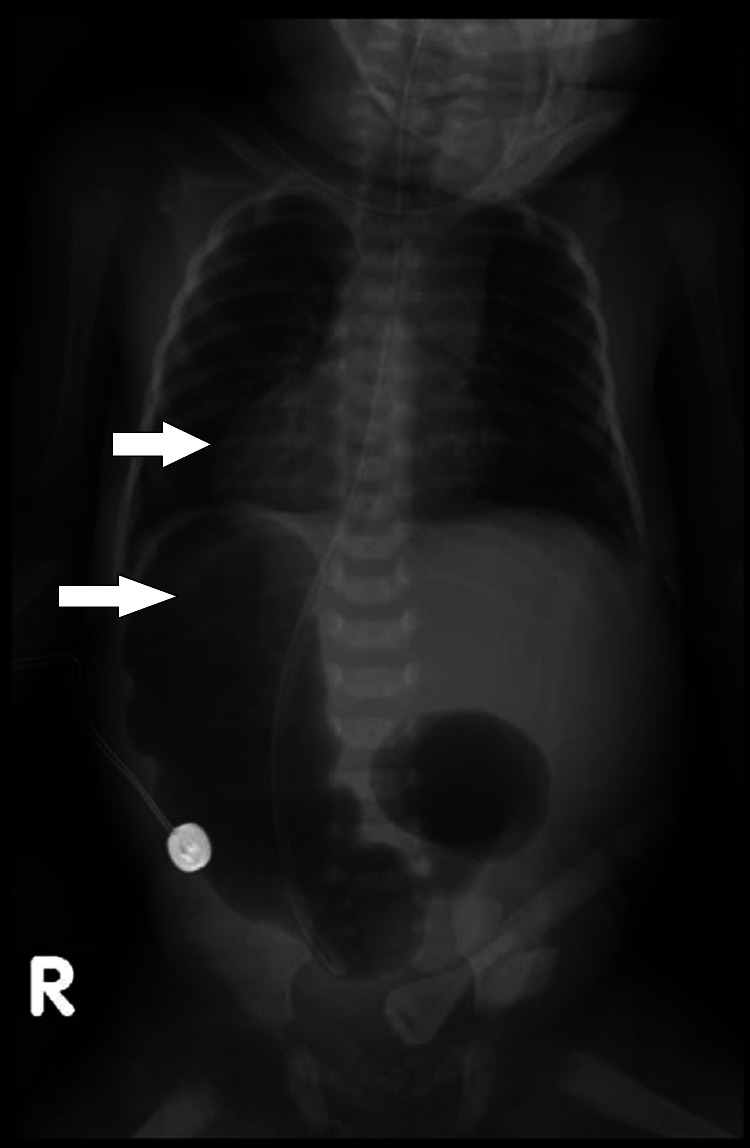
Plain X-ray of chest and abdomen revealing right-sided cardiac shadow, NGT in the stomach with double bubble sign on the right side, and hepatic shadow on the left side. A temperature probe on the right side of the abdomen was used as a site marker. NGT, frequent nasogastric tube.
